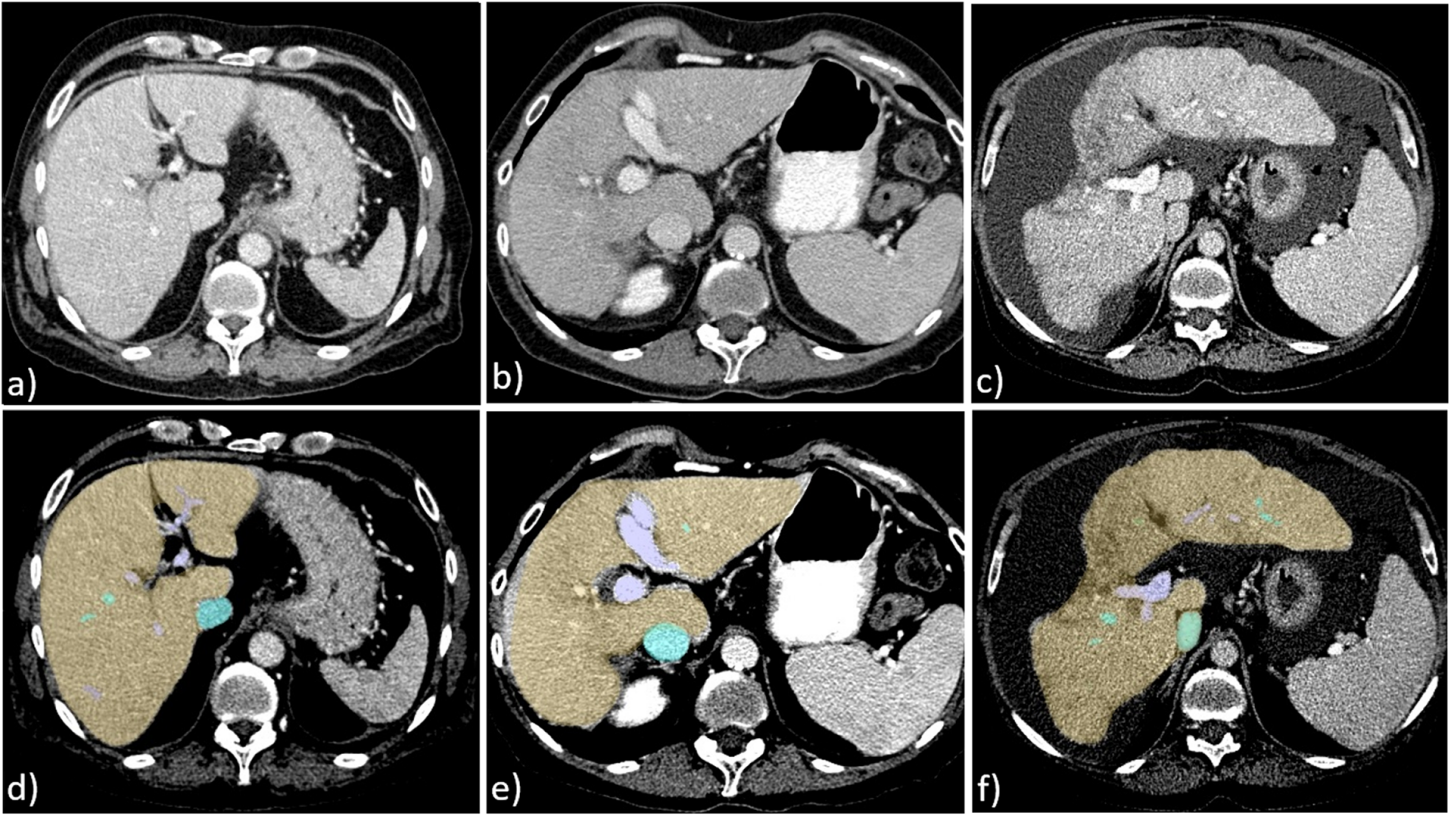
Multiphase computed tomography of three patients. a–c Transversal reconstruction of three patients in portal-venous phase. d–f Pre-processing with semiautomatic liver and vein delineation. a, d Patient with Child-Pugh class A: no changes in liver size or liver parenchyma were observed; all models rated the liver as Child-Pugh class A. b, e Patient with Child-Pugh class B: slight changes in liver configuration as well as heterogeneity of liver parenchyma were observed; only the convolutional network and the expert radiologists’ prediction rated the liver correctly as Child-Pugh class B, whereas the linear regression and the random forest rated it as a Child-Pugh class C. c, f Patient with Child-Pugh class C: overall appearance of the liver exhibits characteristic changes (liver configuration, size, and parenchyma texture); all models rated the liver as Child-Pugh class C.
